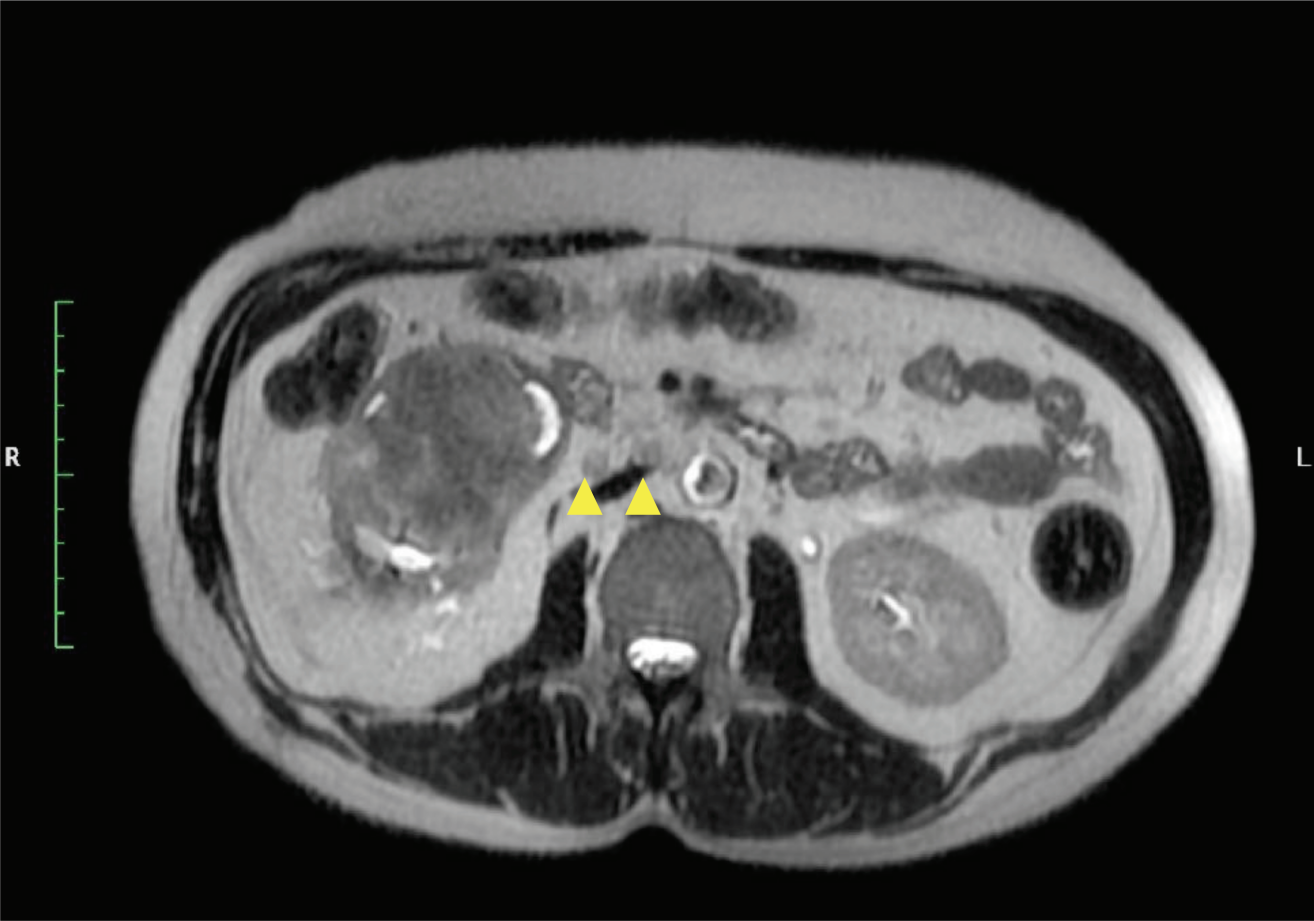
MRI T2 axial image show right renal hilar nodes (yellow arrow heads).
